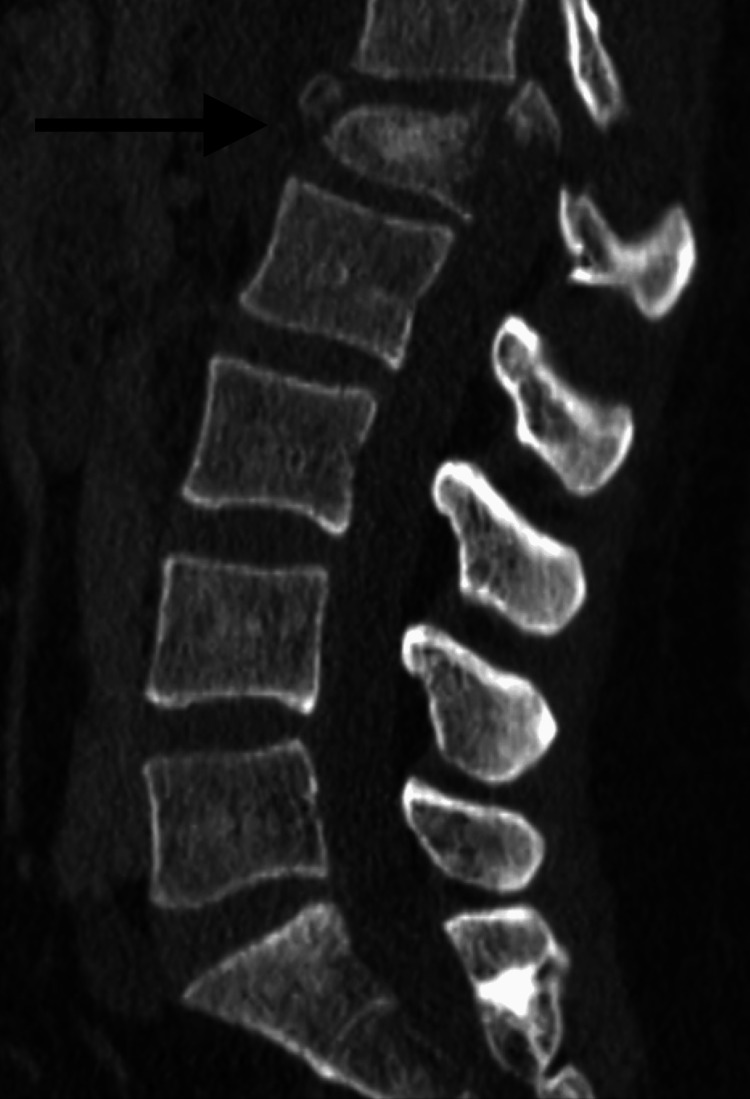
Preoperative sagittal CT of patient 4 demonstrates the complete burst fracture (AOSpine subtype A4) of L1 (black arrow) with significant bony retropulsion into the canal.
